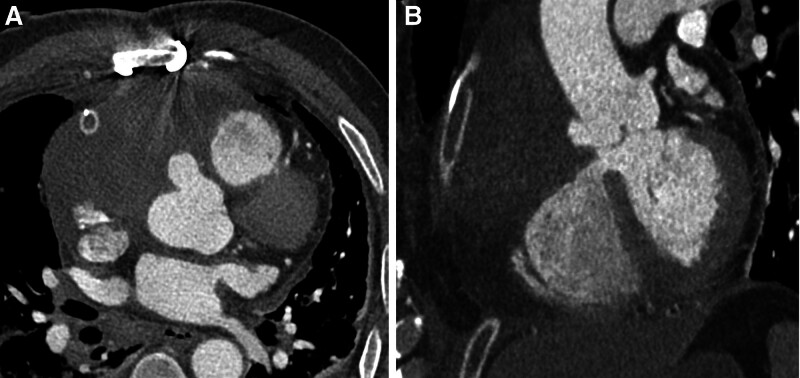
Postoperative chest CTA images of the patient. Postoperatively, the pseudoaneurysm’s rupture site was successfully sealed with a pericardial patch. CTA = computed tomography angiography.
